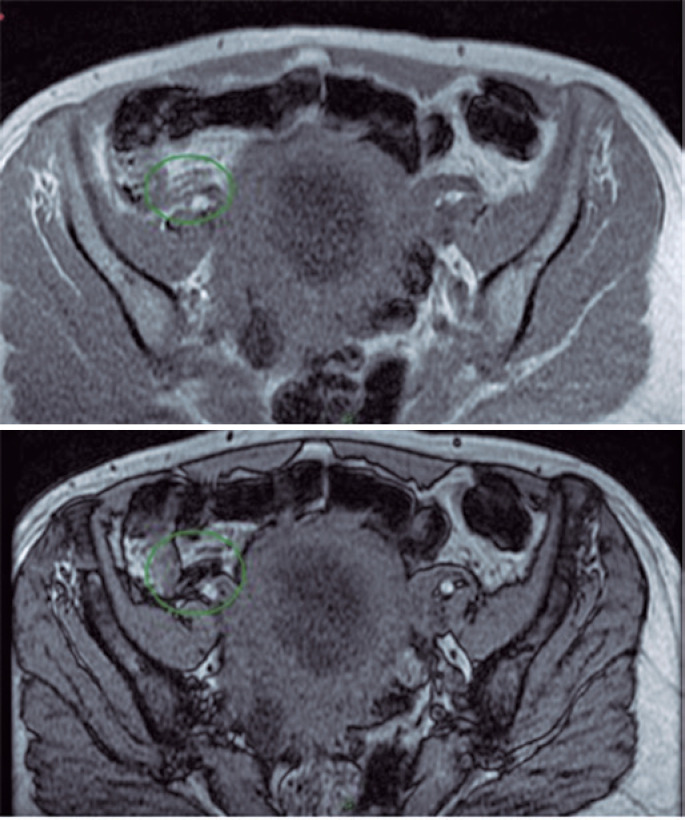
Cecal appendix with thicker wall showed in the axial plan magnetic resonance imaging
